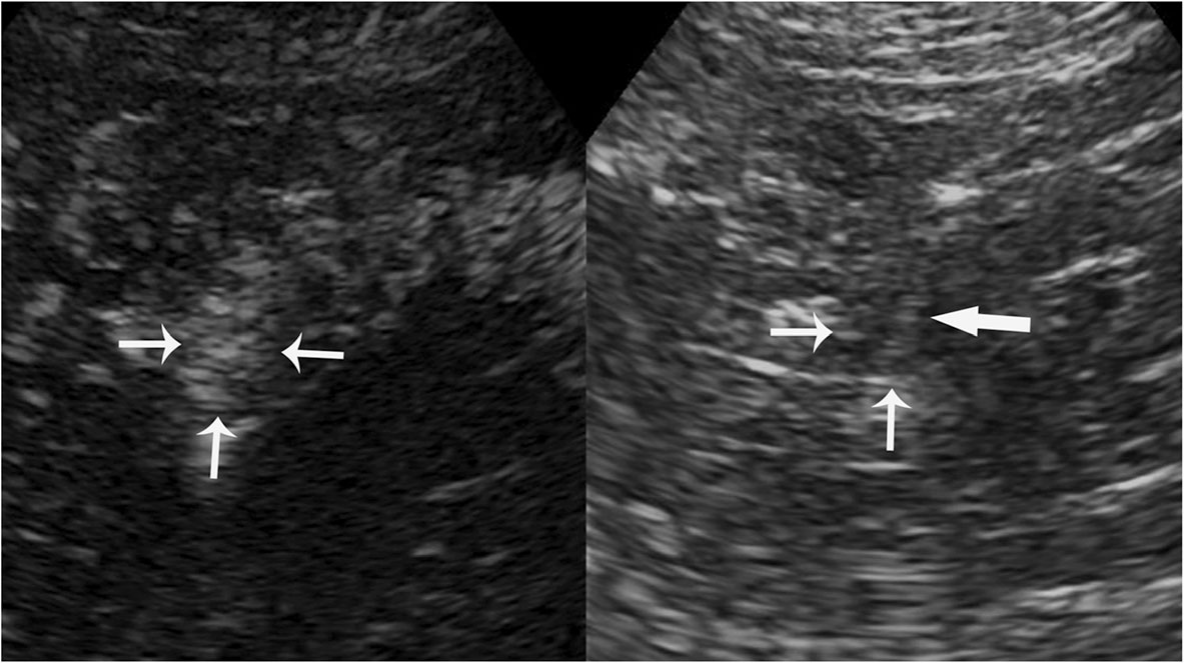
Contrast-enhanced ultrasonography guided biopsy of the mass. The needle tip (wide arrow) has correctly been inserted into the completely enhanced mass (narrow arrow)
